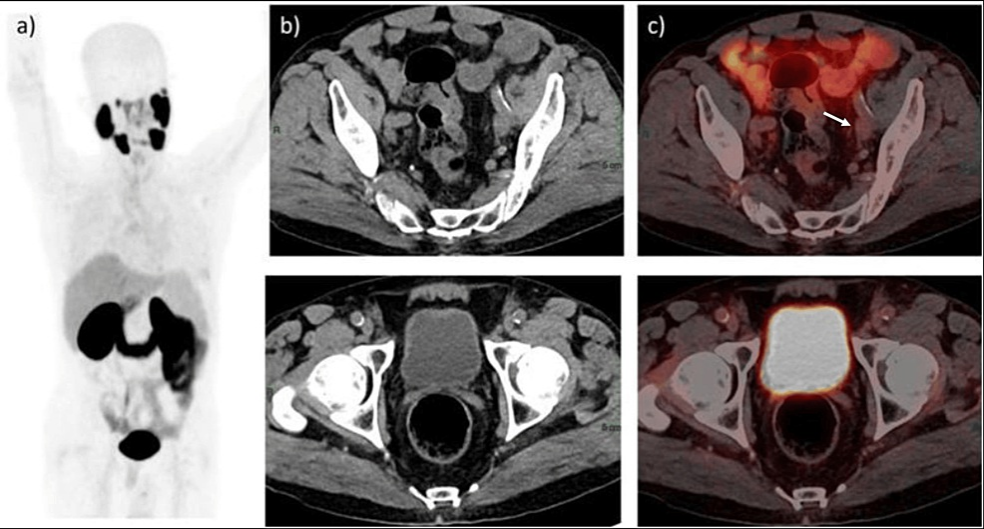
Post-therapy gallium-68-prostate-specific membrane antigen positron emission tomography/computed tomography (68Ga-PSMA-PET/CT). (a) Maximum intensity projection image. (b) Non-contrast CT. (c) Fused PET/CT images show complete resolution of prior PSMA avid seen in the prostate gland extending to the seminal vesicle. No abnormal uptake is seen in the prostate. Near-complete resolution of prior 68Ga-PSMA-avid perirectal and bilateral external iliac lymph nodes. Small bilateral external iliac lymph nodes are seen with no significant tracer uptake, with the largest measuring 1.3 x 1.0 cm at the left external iliac region (white arrow).
